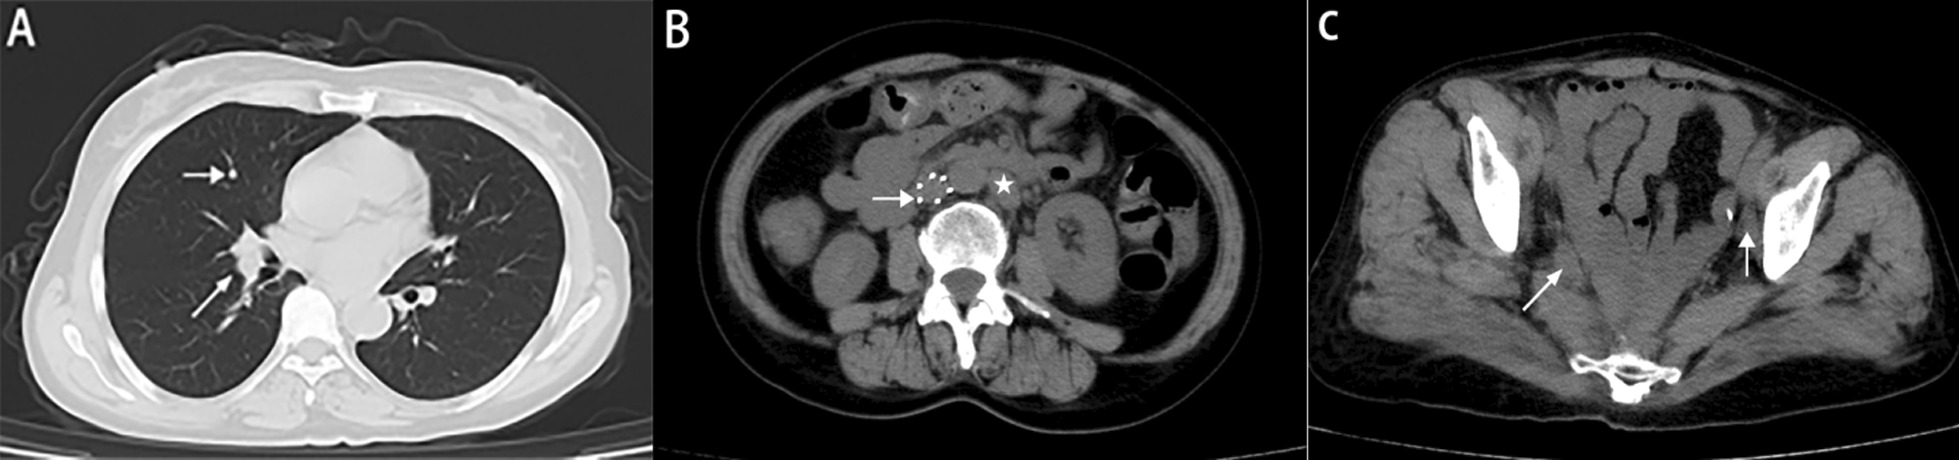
Imaging data from October 2021 A CT showed new metastatic nodules in the right lung (arrow). B Retroperitoneal enlarged lymph nodes are located on the left side of the aorta (asterisk), and a filter in the Inferior vena cava (arrow). C The pelvic nodules were stable (arrow)
